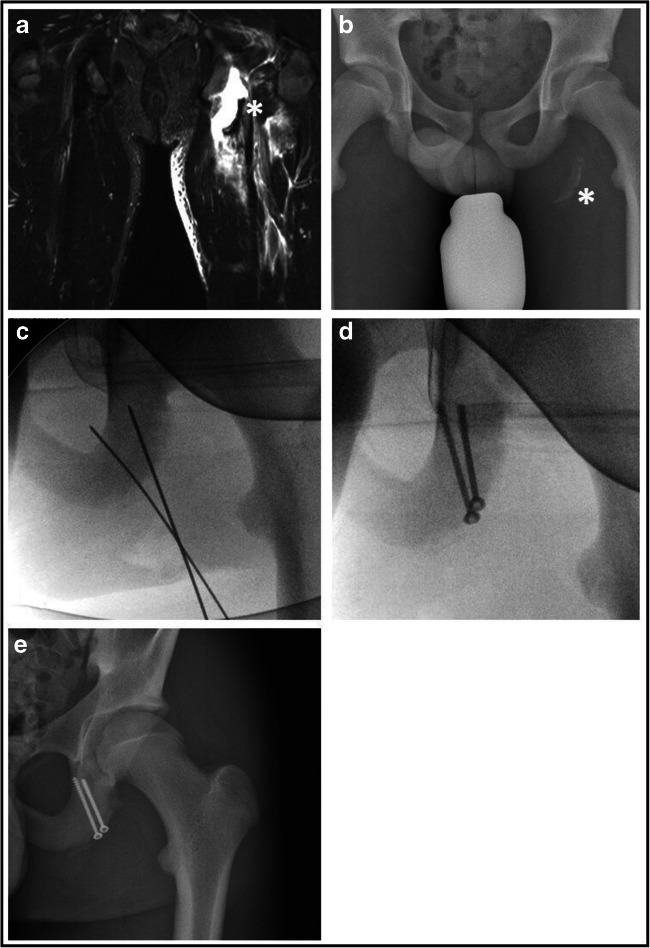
Refixation of acute left-sided avulsion fracture one week after trauma (a: coronal MRI picture, b: plain pelvis radiograph, c: intra-operative temporary fixation via guiding k-wires, d: intra-operative control after refixation with cancellous bone screws, e: post-operative control on plain radiographs, star*: bony fragment)
